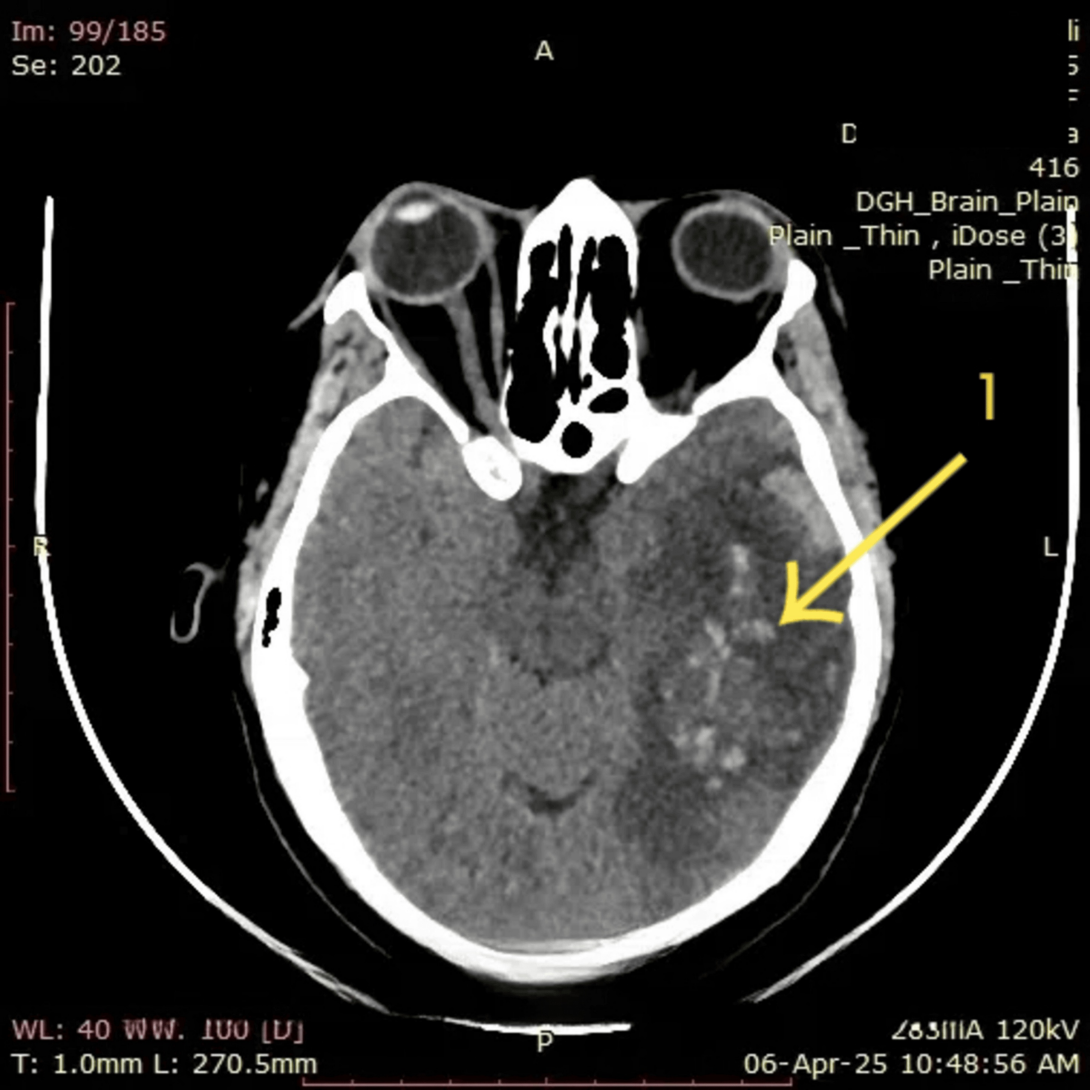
Non-contrast CT brain (05/04/2025) Arrow 1: The hemorrhagic focus is surrounded by extensive hypodense edema, consistent with venous infarction.
